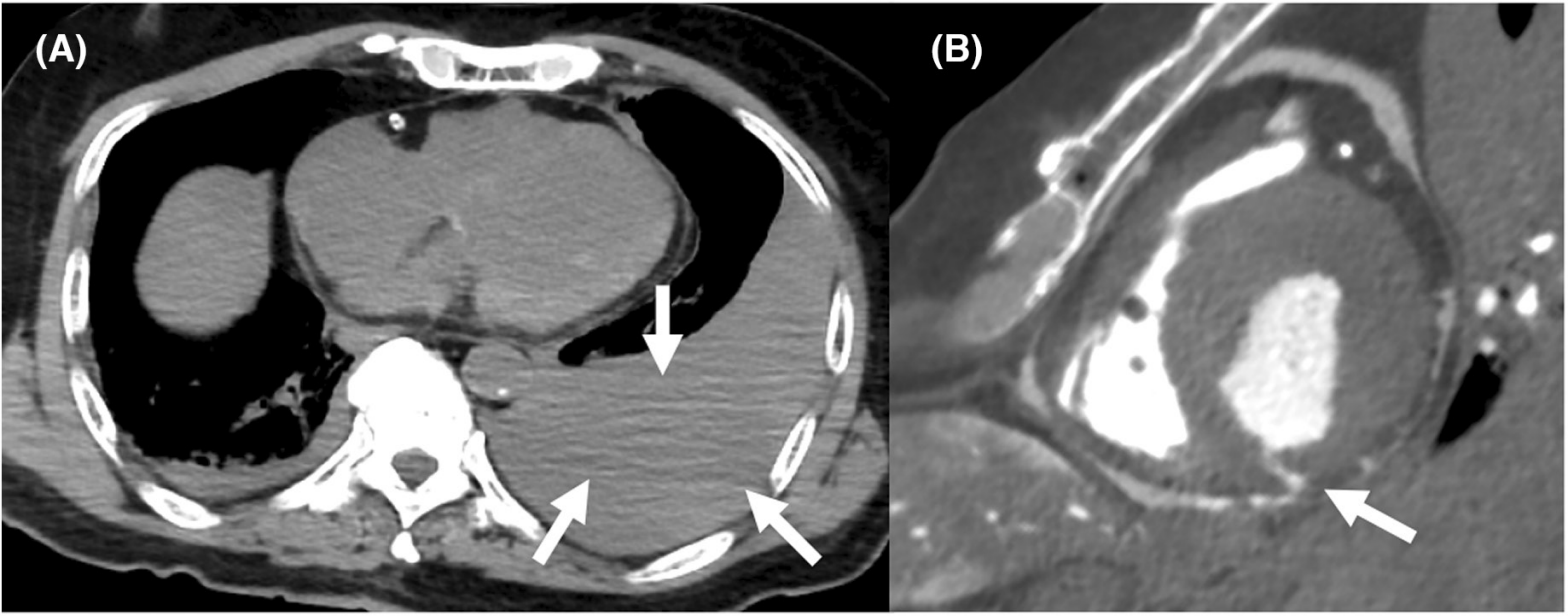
Autopsy imaging (AI). (A) Plain CT shows massive pleural effusion (arrows) of 50 HU on AI, which implied that the fluid was blood. (B) After a few sets of chest compression, CT showed the contrast agent flow (arrow) from the cardiac cavity into the pericardium. AI, autopsy imaging; CT, computed tomography; HU, Hounsfield unit.
